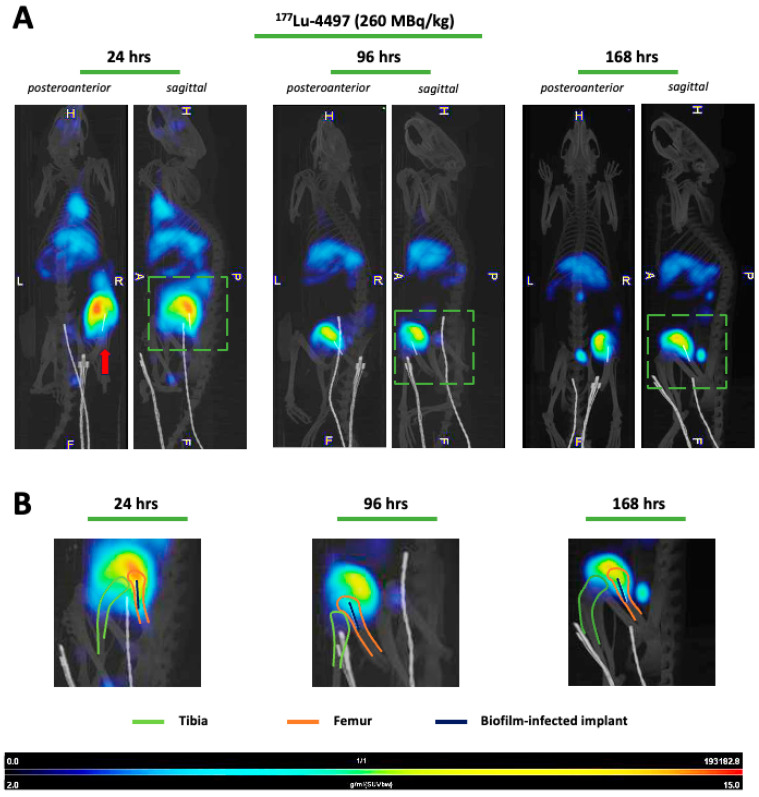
SPECT/CT analyses with ^177^Lu-4497 (260 MBq/kg) show specific accumulation after 24, 96 and 168 h. Posteroanterior and sagittal planes are depicted. H = head, L = left-side, R = right-side, P = posterior side, A = anterior side, and F = feet. The red arrow indicates the biofilm-infected implant site. The green square highlights the close-up shown in section B. (A) With the 4497-IgG1 antibody (anti-β-GlcNAc WTA) labeled with Lutetium-177, specific bacterial targeting and uptake are seen across all timepoints. (B) Close-up of the sagittal plane displaying the position of tibia, femur and biofilm-infected implant. Most accumulation is observed in the knee joint and articular capsule, distal to the implant infection.
